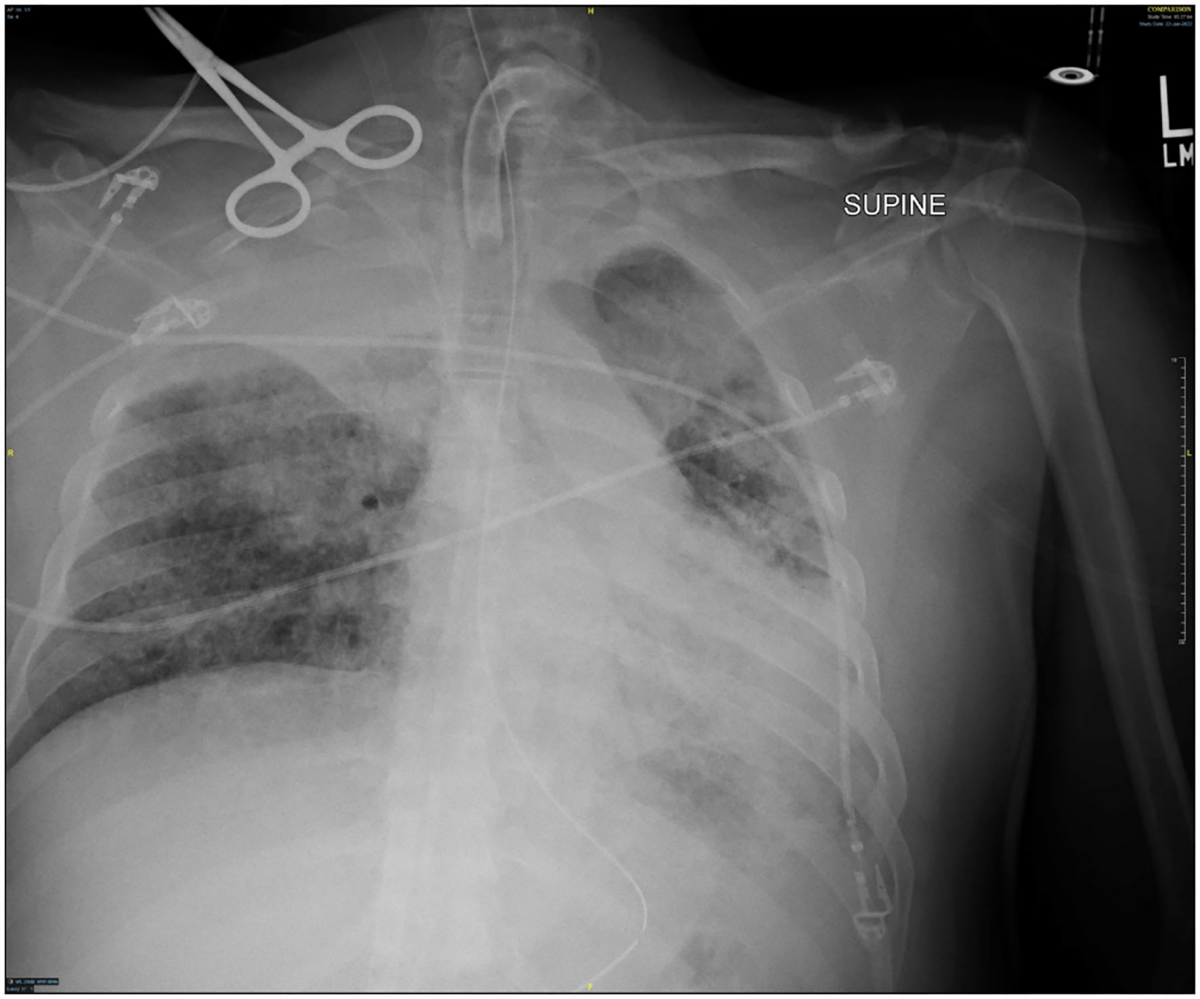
Chest x-ray 4 days after resuming ventilator.
